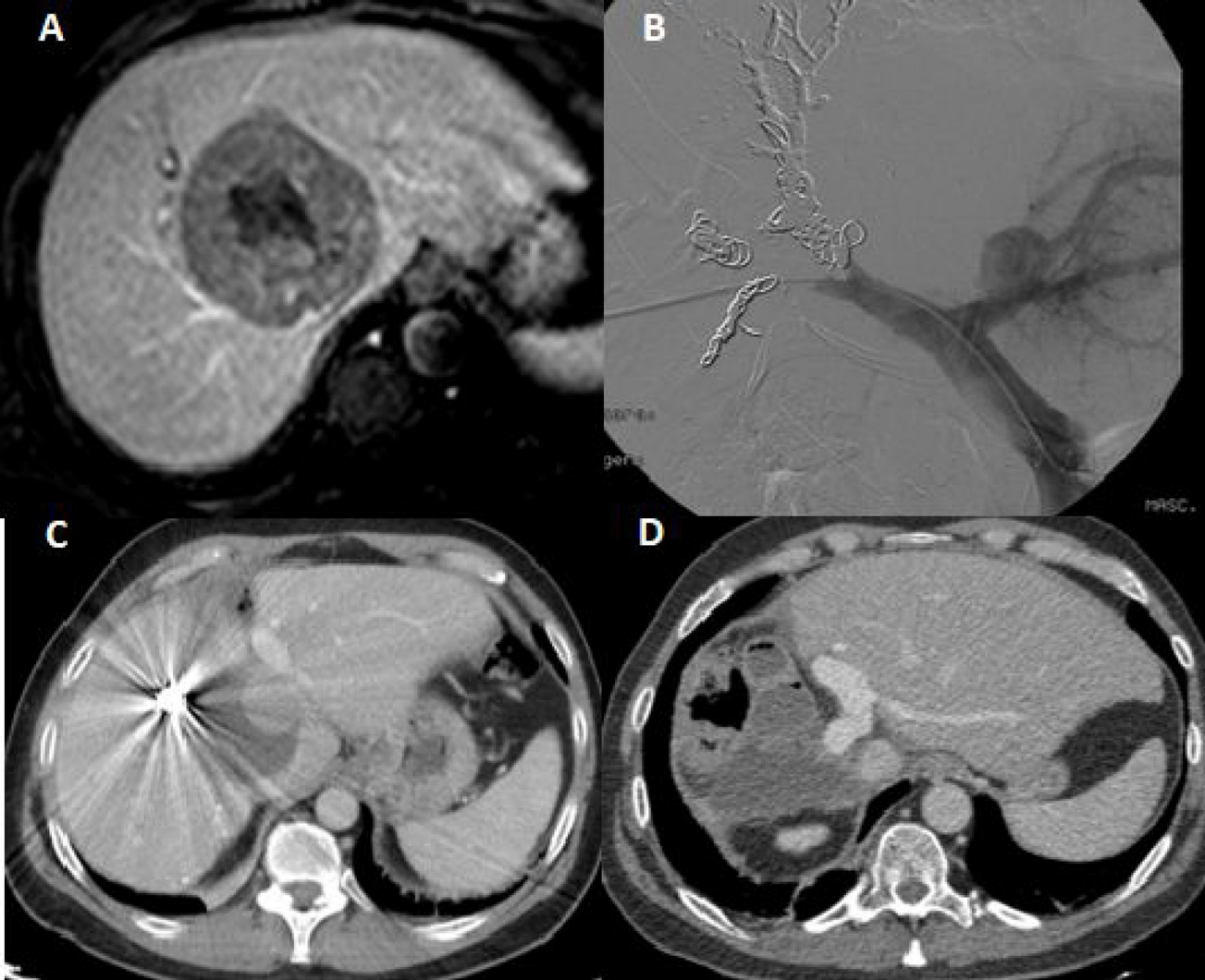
(A) Very large HCC with hepatitis B viral cirrhosis involving the right hepatic lobe. CT image depicting very large HCC involving the middle and right hepatic vein. (B) On day 4 after tourniquet placement during the first stage, we performed a right portal vein embolization. (C) CT volumetry on day 9 after stage I showing a future liver remnant increase of 105%. (D) Follow-up CT at 4 months shows the disease-free liver after right trisectionectomy.
